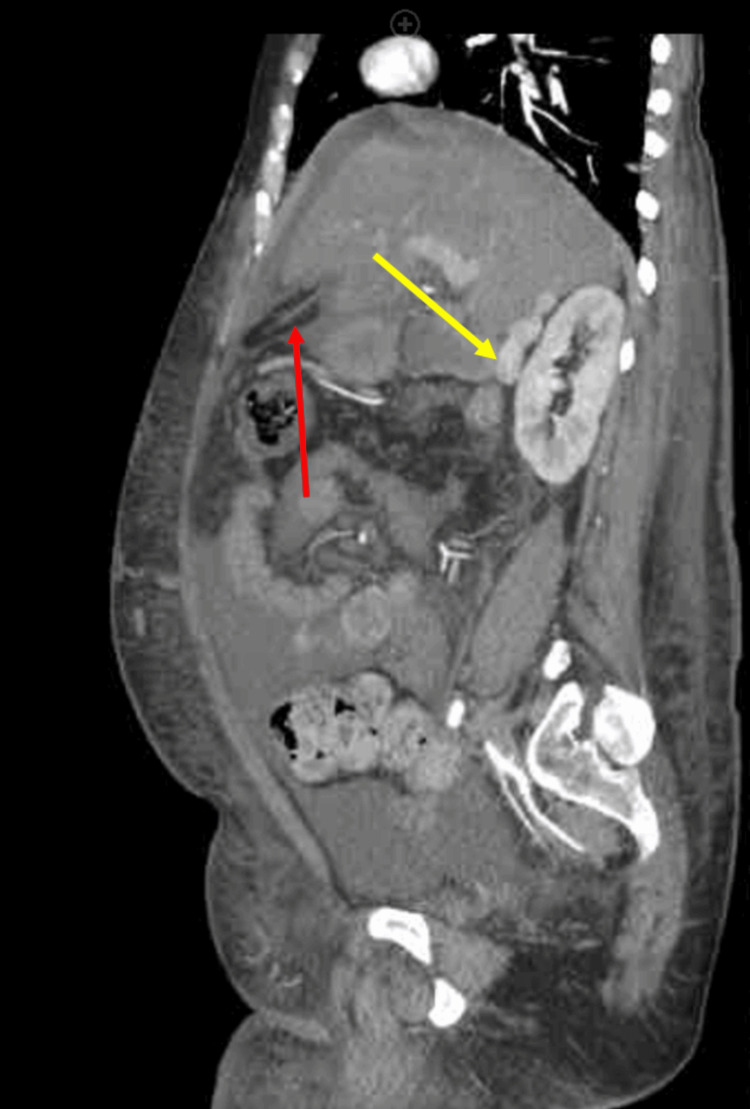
Sagittal abdominal/pelvis CTA Demonstrates recanalization of the umbilical vein from IVC occlusion, highlighted by a red arrow. A yellow arrow denotes a supra-renal IVC. A hypoplastic infrarenal IVC is observed below this point. CTA: computed tomographic angiography; IVC: inferior vena cava
